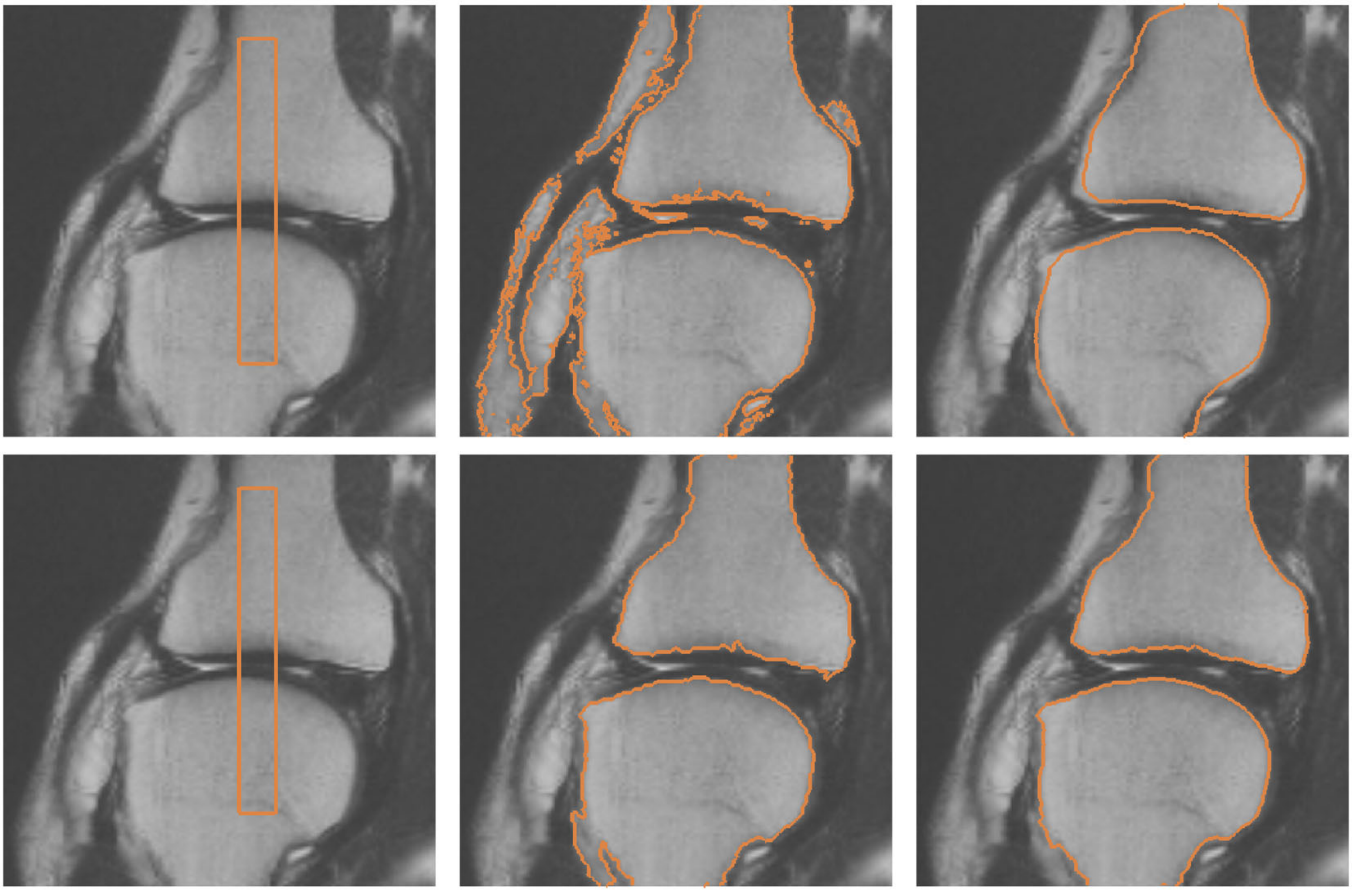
Segmentation of the knee from magnetic resonance image: (top row, from left to right) initial contour, Chan-Vese model, Chan-Vese model with shape prior, (bottom row, from left to right) initial contour, gradient vector interaction-based energy, gradient vector interaction, and shape-based energy.
